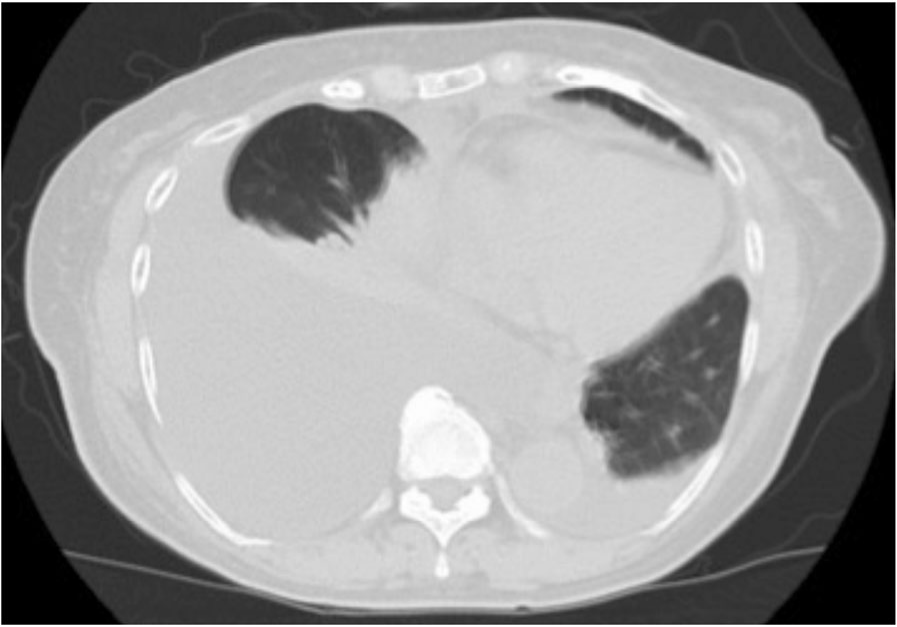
Computed tomography showing large pleural effusion on postoperative day 42
